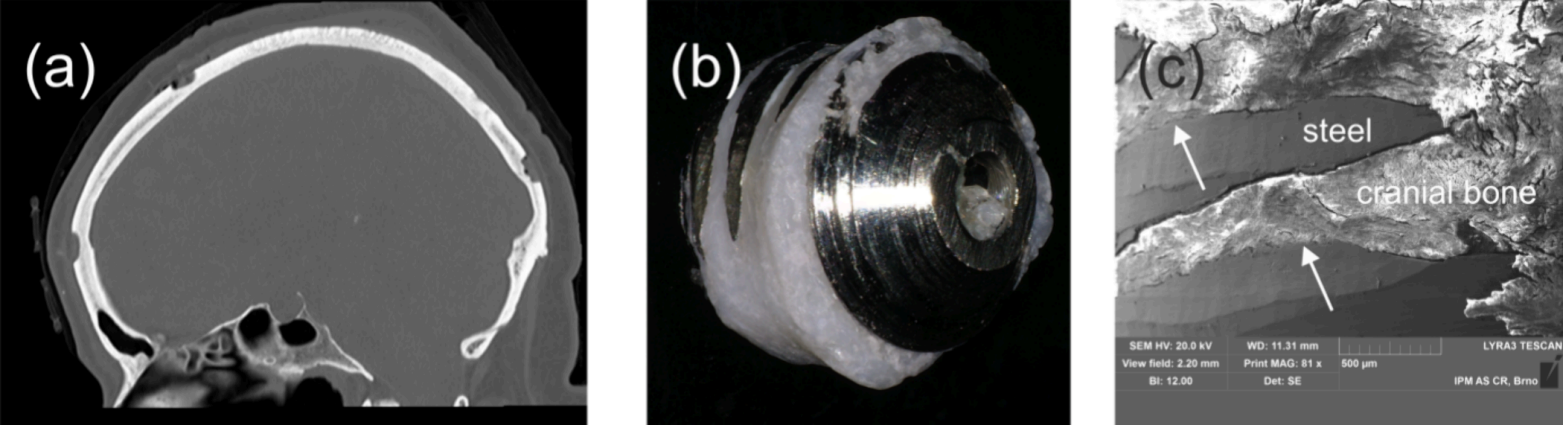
(a) Postoperation CT image documenting
